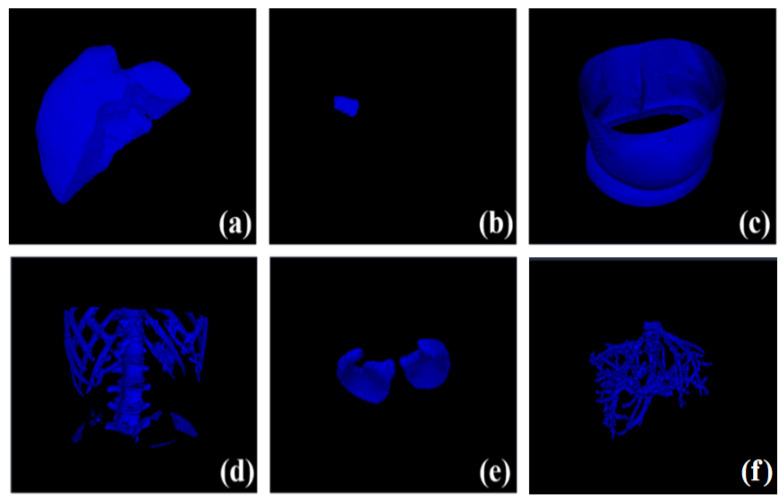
Isosurface rendering results of critical structures segmented in CT image by our system. (a–f) Volume rendering of segmented liver, liver tumor, skin, bone, lung, and liver vessel.
